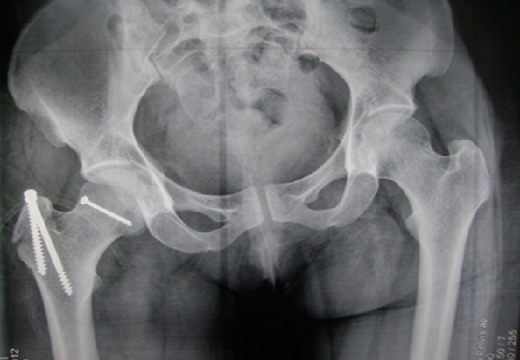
Radiographie du bassin de face en post opératoire immédiat, objectivant l'ostéosynthèse du fragment céphalique et de la trochantérotomie
